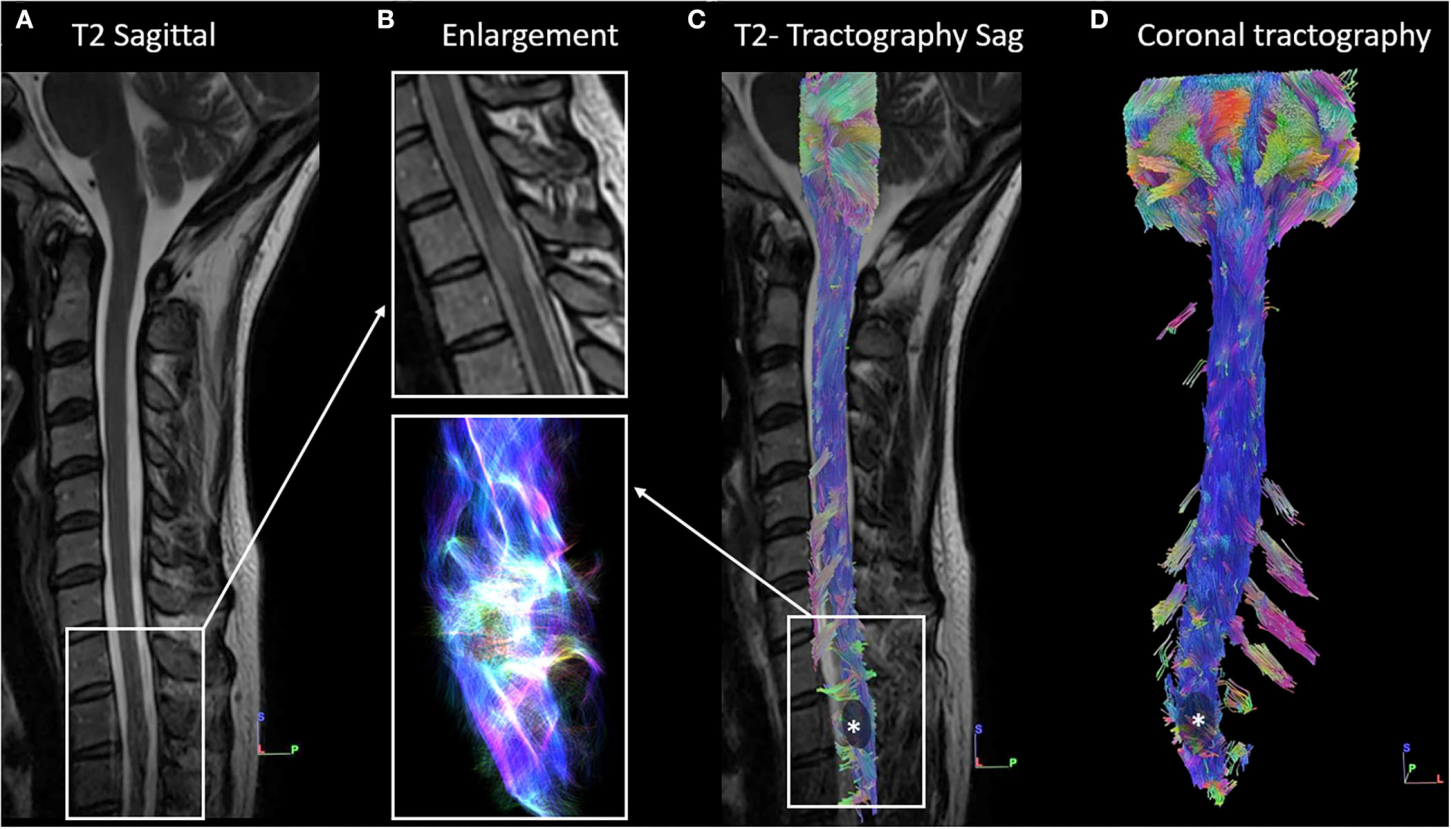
Full cervical cord tractography in a patient with intramedullary tumor. (A) Sagittal T2-weighed image showing intramedullary isosignal at the Th2-Th3 spine level, associated with an increase in the volume of the spinal cord. (B) 1-Enlargement of the (A) at the level of intramedullary lesion, on the T2-weighed image. 2- Enlargement of the (C) on fiber tracking, according to a “line” style rendering performed in DSI Studio, at the level of the lesion. It shows warped fibers within the tumor, without safe access for spinal cord surgery. (C) Overlay of the spinal cord tractography on the sagittal T2-weighted image confirmed fiber deformation at the Th2-Th3 level. (D) Ventral view of spinal cord tractography showing warped fibers at the level of the tumor (in black with *).
